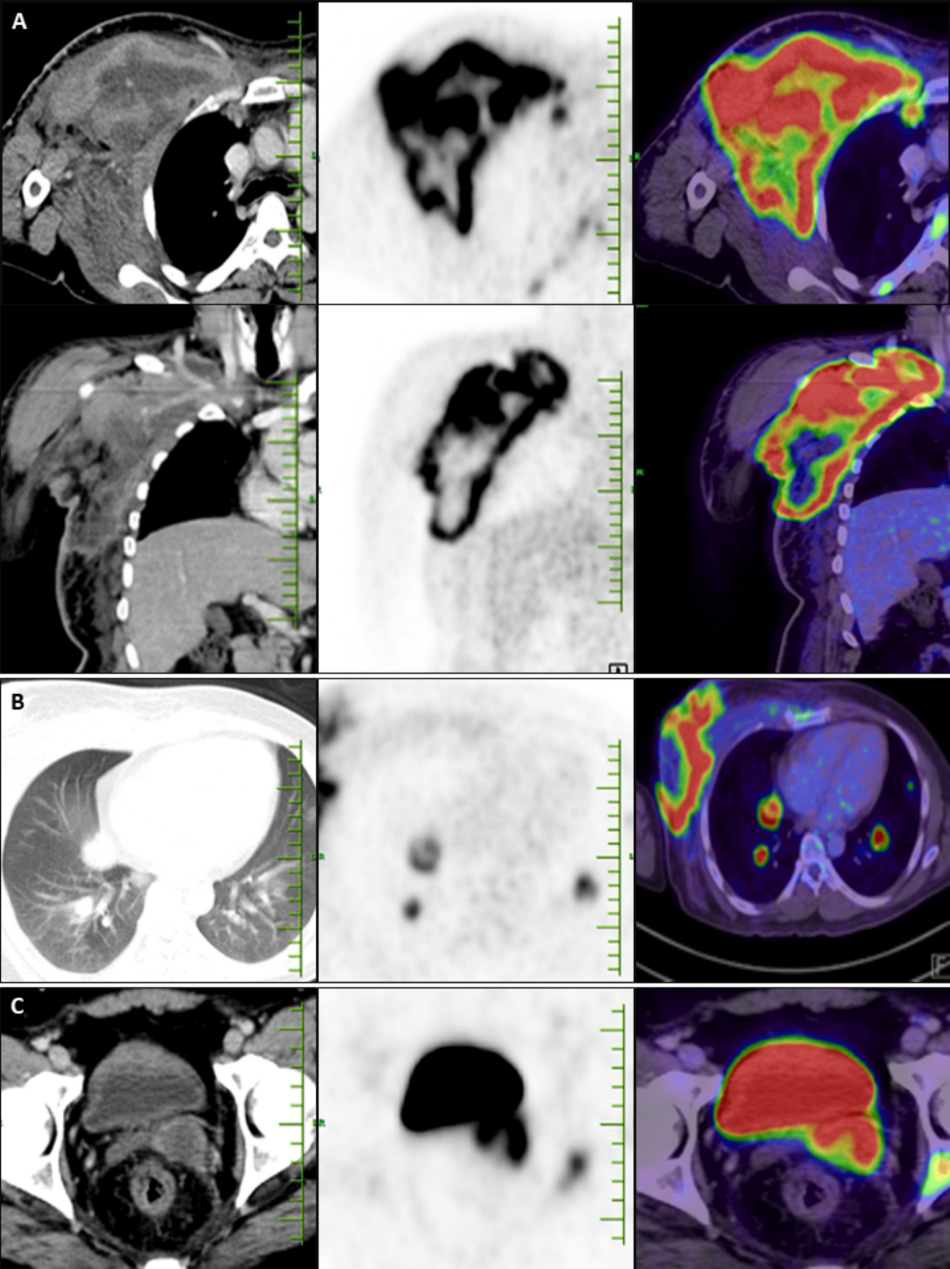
PET-CT Images Before Starting Chemotherapy. A large, ill-defined, geographically shaped FDG avid (SUV max 18.8) necrotic mass lesion was seen involving the muscular plane of the right anterolateral chest wall, measuring ~14.0 × 17.5 × 19.5 cm (AP × TR × CC); (B) Multiple FDG avid (SUV max 7.5) nodules seen in bilateral lungs, the largest measuring 29 × 22 mm in the right lower lobe; (C) FDG avid (SUV max 13.4) hypoenhancing lesion seen in the left seminal vesicle, measuring 32 × 23 mm-Metastatic deposits. FDG: fluorodeoxyglucose
